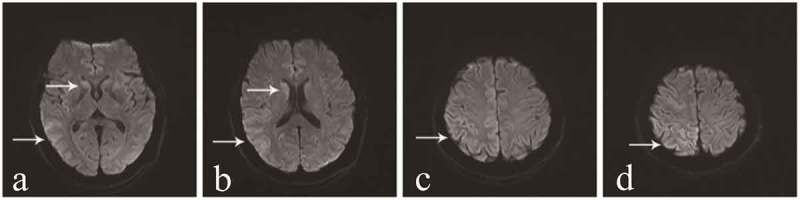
Results of DWI scans. a  and b  show an increase in signal intensity changes at the surface of the temporal lobe and bilateral basal ganglia regions (especially the caudate nucleus). c  and d  show ribbon-like signals in the right parietal lobe.
